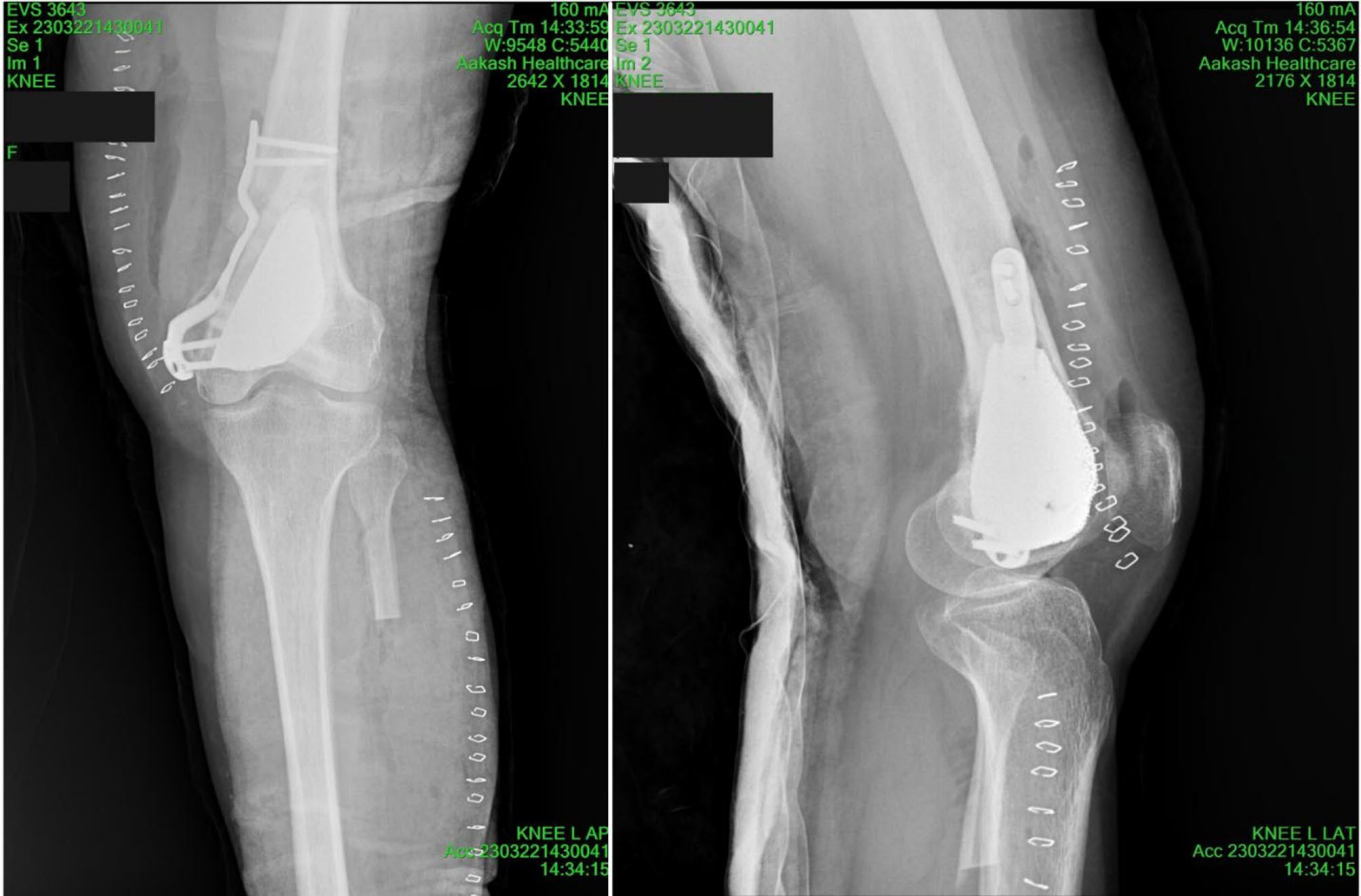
Post-operative radiograph of the left femur. X-ray image taken post-op immediately after surgery, showing the surgical site, after suturing and bandaging, displaying the surgical site post-procedure
